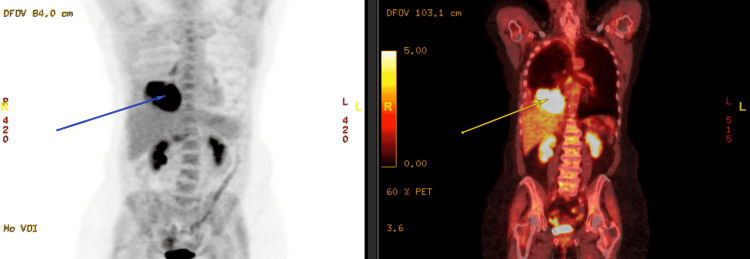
PET-CT scan coronal section demonstrating a metabolically active right lower lobe heterogeneous mass (arrows) PET: positron emission tomography
